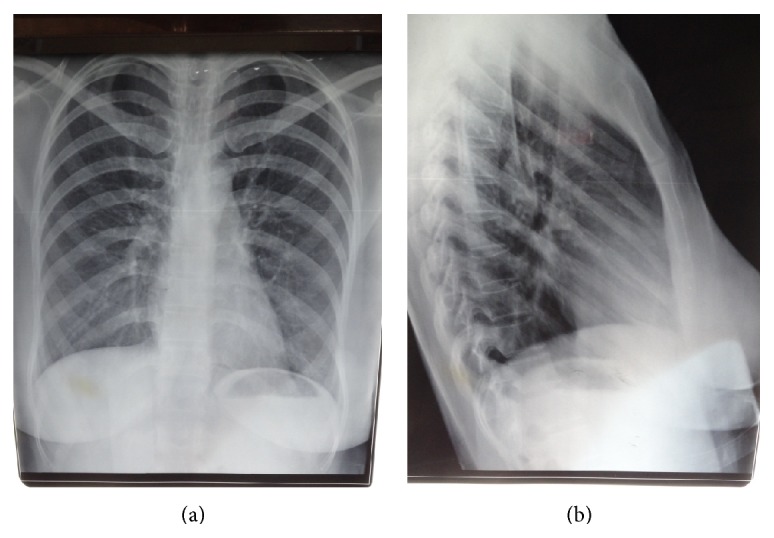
Posteroanterior and lateral chest radiograph, respectively, showing clear lung fields after completion of six-month antituberculous medication.
